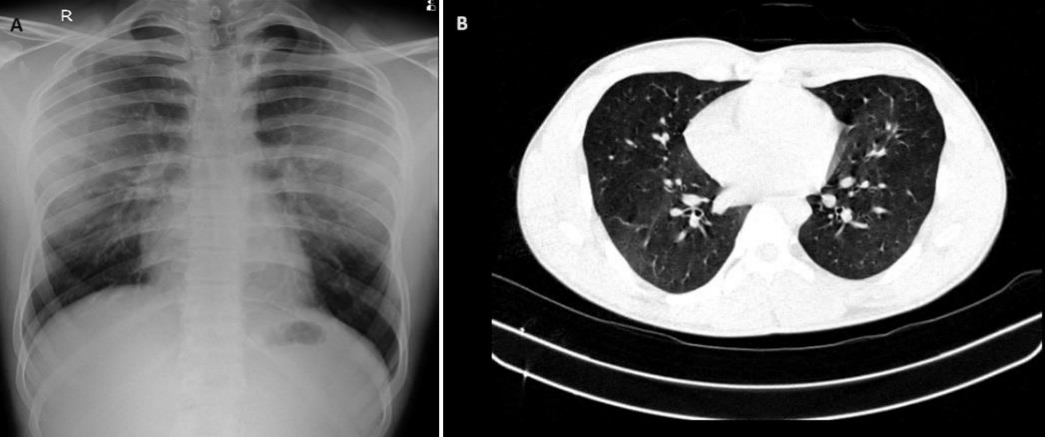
A) chest Xray showing bilateral non homogenous opacity; B) high resolution CT thorax showing bilateral ground glass opacification with centrilobular nodules
